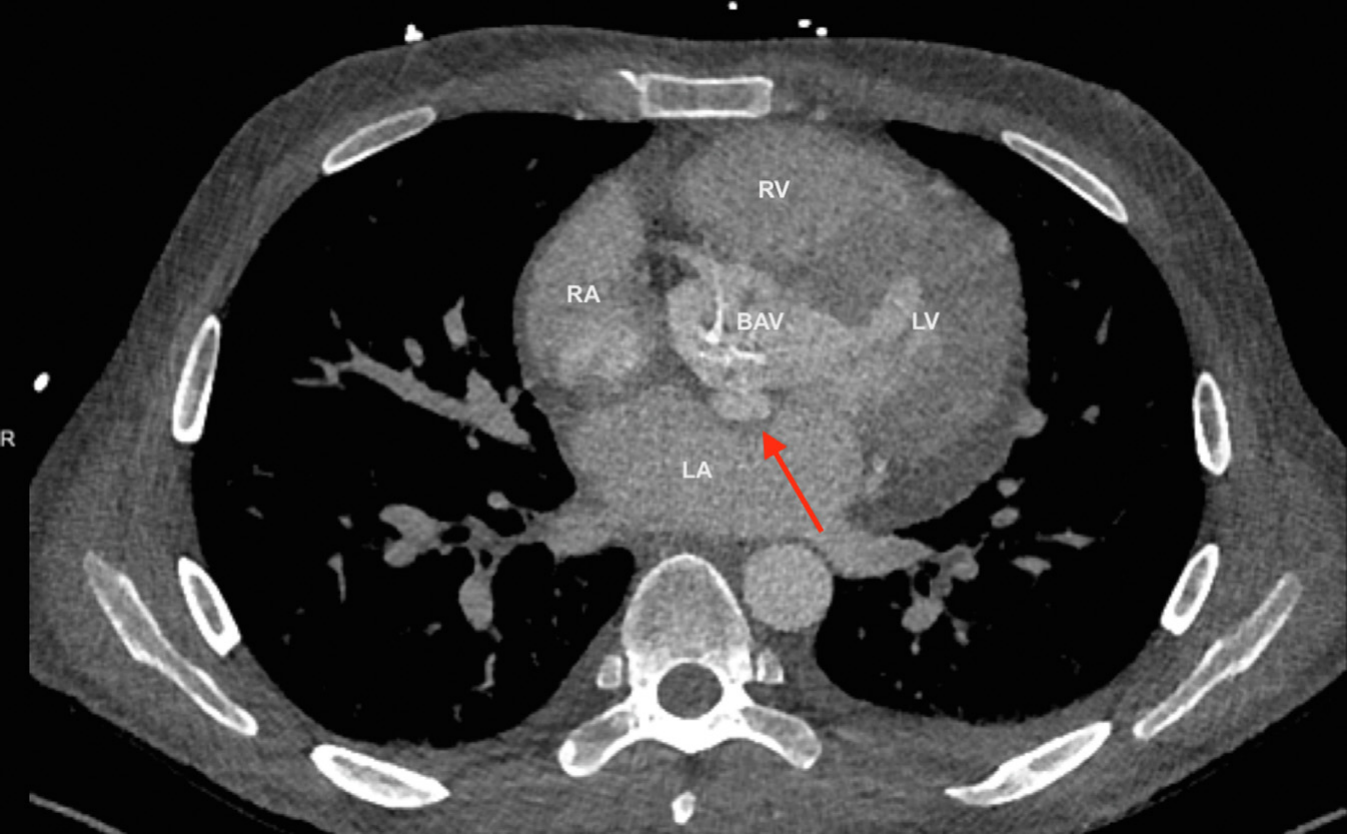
Computed Tomography Angiography of the Chest CT angiography of the chest showing an aortic root pseudoaneurysm (red arrow) arising just inferior to the junction of the noncoronary cusp and left coronary cusp projecting toward the aortomitral curtain. BAV = bicuspid aortic valve; LA = left atrium; LV = left ventricle; RA = right atrium; RV = right ventricle; other abbreviations as in Figure 1.
